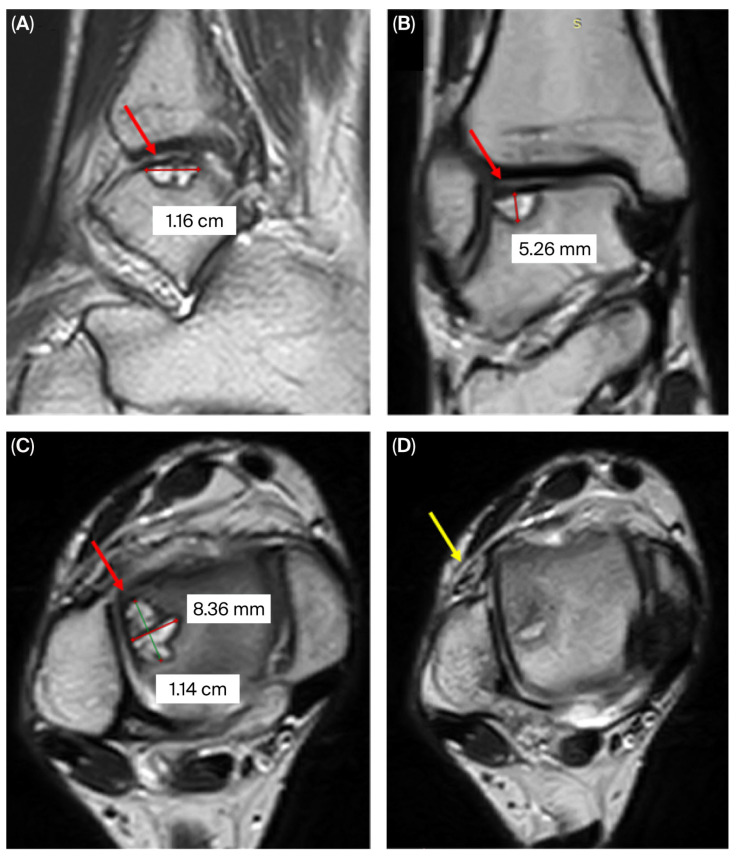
MRI of the right ankle joint showing the osteochondral lesion in the lateral talar dome (red arrow): (A) sagittal, (B) coronal, (C) axial; and an anterior talofibular ligament injury (yellow arrow) (D).
